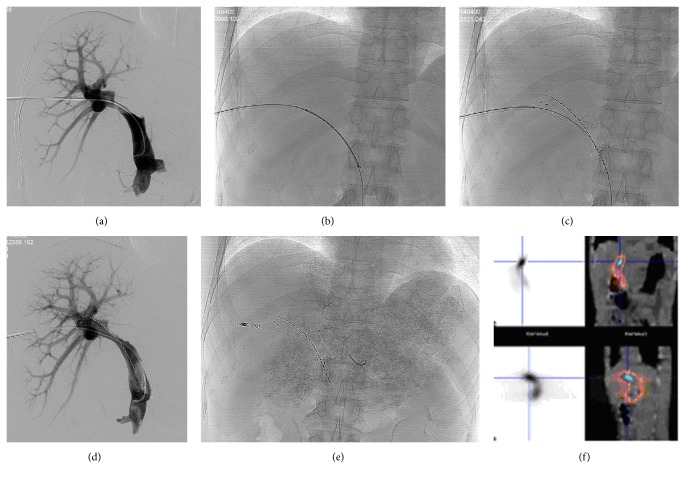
Images of ^125^I seed strand implantation in a 53-year-old man. (a) After the patent 2nd-order branch of the left portal vein was catheterized, a 5-F Cobra catheter was placed in the main portal vein (MPV). Tumor thrombus in the proximal MPV and sagittal segment of the left portal vein was clearly shown in the right anterior oblique portography projection. (b) Transcatheter implantation of ^125^I seed strand. (c) Under guidance of the second guide wire, the portal vein stent was implanted. (d) Angiography after vein stent and ^125^I seed strand implantation showing the portal vein blood flow and that the seeds position is good. (e) A 14 × 120-mm self-expandable stent and ^125^I seed strand with 20 seeds were placed precisely in the obstructed MPV. The ^125^I seed strand was fixed steadily between the stent and MPV. Good flow through the patent stent from distal MPV to left portal vein is shown on the portography. (f) Images from single-photon emission computed tomography (SPECT)/computed tomography (CT) 1 day after procedure. The stent and ^125^I seed strand were placed correctly, without displacement. Radiation emitted by the ^125^I seed strand was distributed homogeneously. It presented as a cylindrical shape with a diameter of 20 mm covering the target lesion completely.
